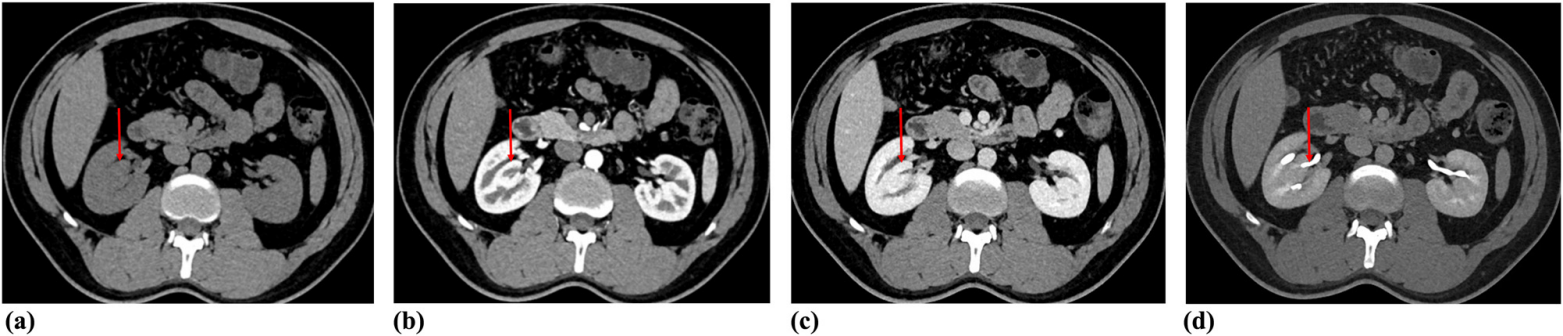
Axial precontrast (a) and contrast-enhanced (b and c) CT showed oval solid mass (red arrows) in right renal sinus with identical density and enhancement pattern to adjacent normal renal cortex. In pyelographic phase image (d) exhibited the small renal pelvis (red arrow) from the pseudo-mass merging into the right renal pelvis.
